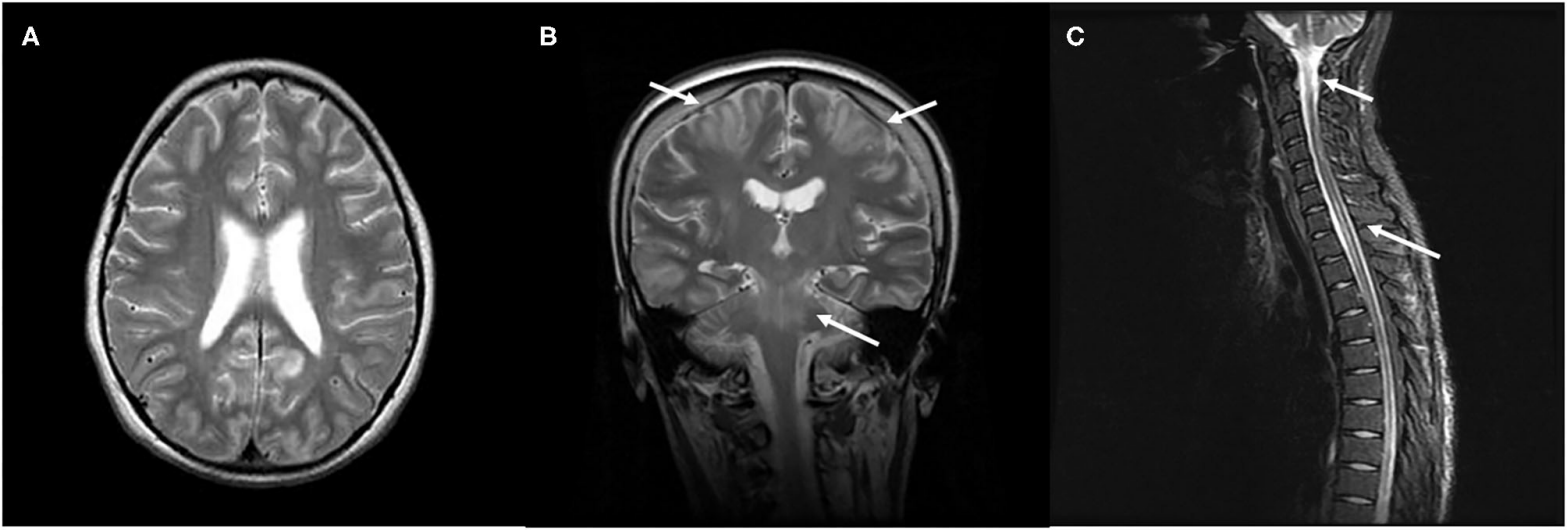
Characteristic neuroimaging findings of a 15-year-old male with posterior reversible encephalopathy syndrome with spinal cord involvement (PRES-SCI). (A) Axial view of brain MRI showing T2-weighted hyperintensity in the bilateral parieto-occipital and frontal lobes. (B) Coronal view of MRI showing T2 hyperintensity in the brain stem, as well as in bilateral frontoparietal lobes (white arrows). (C) Sagittal cervicothoracic spine demonstrating longitudinally T2-weighted hyperintensity originating at cervicomedullary junction (white arrows).
